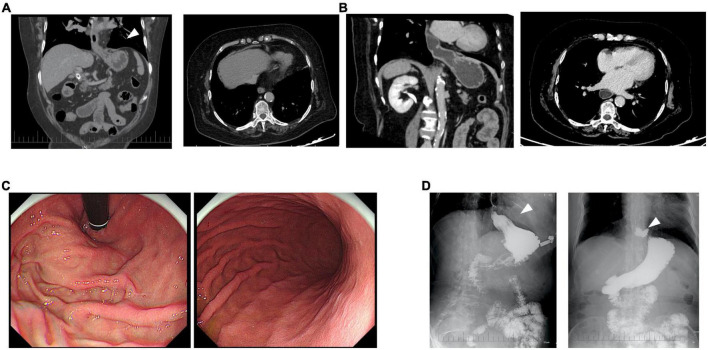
Clinical diagnosis of immune checkpoint inhibitor (ICI)-related esophageal hiatal hernia. At the sixth (A) and eighth month (B) after the onset of immune-related irAEs, a computed tomography (CT) scan for the first time showed abdominal viscera protruding into the left hemithorax (arrow). Upper gastroenterography and endoscopy showed no esophagitis and inflammation of the gastric body and antrum (C), but a clinically confirmed diagnosis of sliding hiatal hernia of the esophagus (type I) was made (arrow) (D).
